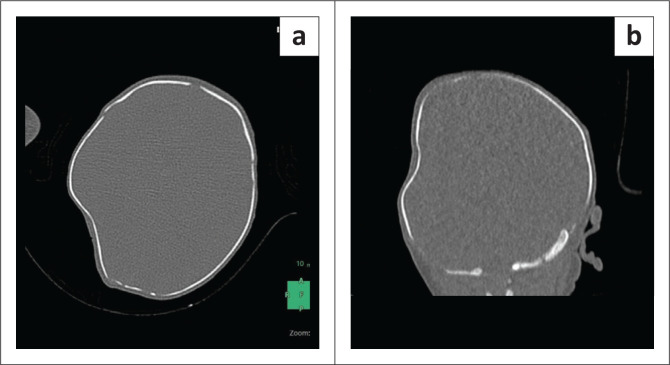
(a) Axial and (b) coronal view of the CT brain scan (bone window).
